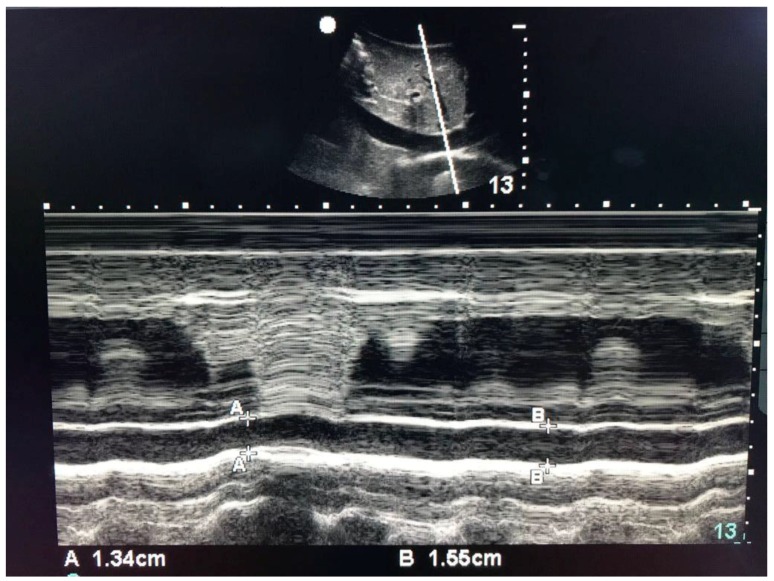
Ultrasound measurements of inferior vena cava (IVC). Panel above shows two-dimensional scan of the IVC with right atrium to the left and panel below shows M-mode scan with respiratory variations in diameter. dIVCmax = maximum diameter of IVC; dIVCmin = minimum diameter of IVC.
